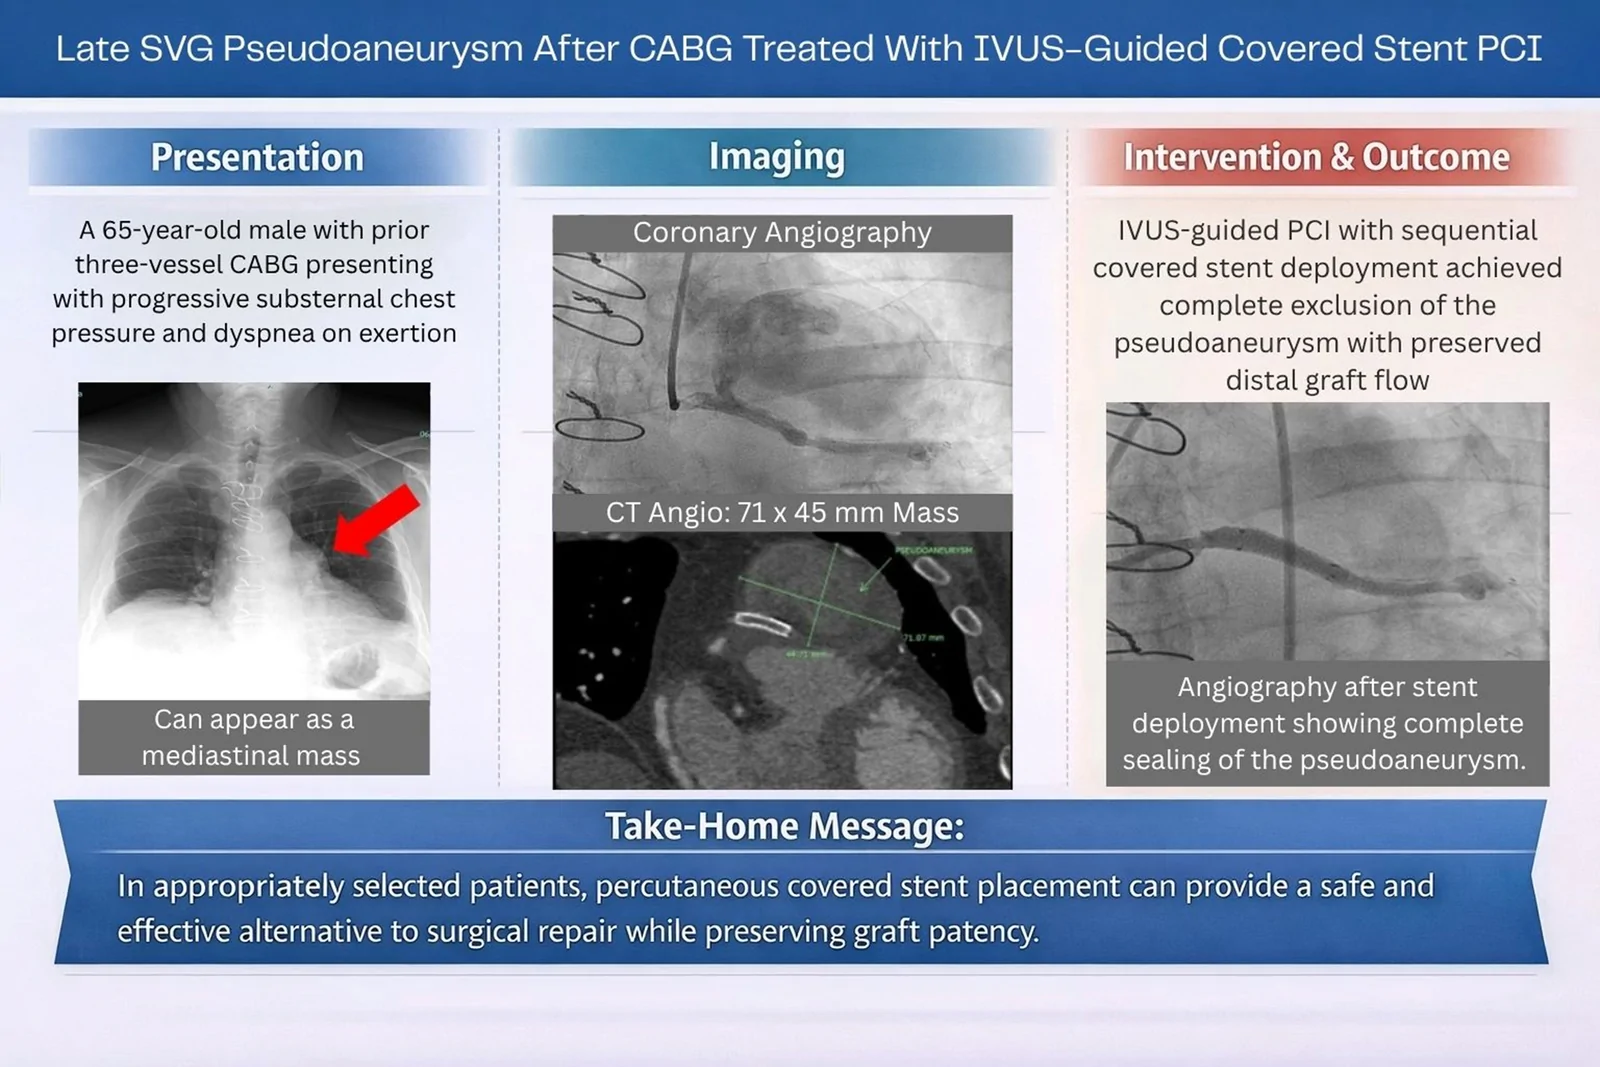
Diagnosis and Percutaneous Management of a Late Saphenous Vein Graft Pseudoaneurysm CABG = coronary artery bypass graft; CT Angio = computed tomography angiography; IVUS = intravascular ultrasound; PCI = percutaneous coronary intervention.
